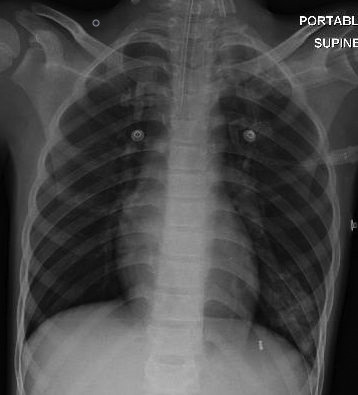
X-ray of the chest showing opacification on the left lower zone.
